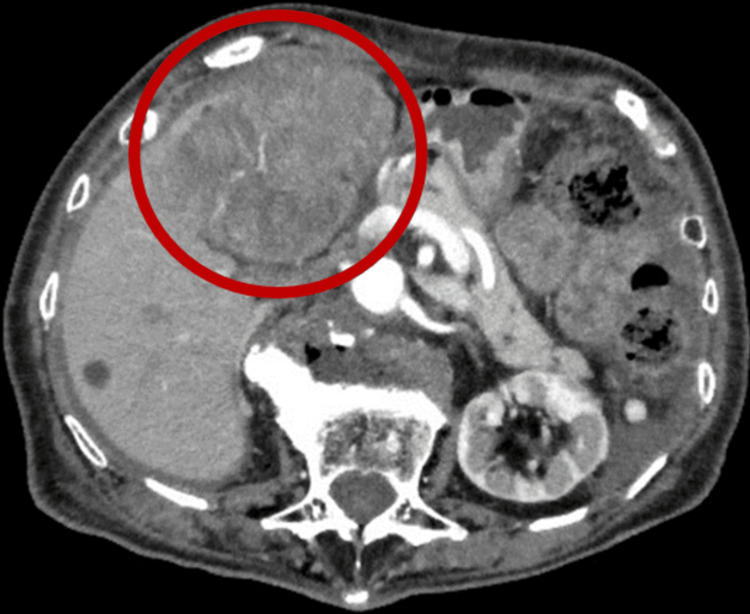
Dynamic CT of the abdomen revealing an 82 × 63 mm hypervascular mosaic mass in the S4 area of the liver (red circle) CT: computed tomography
